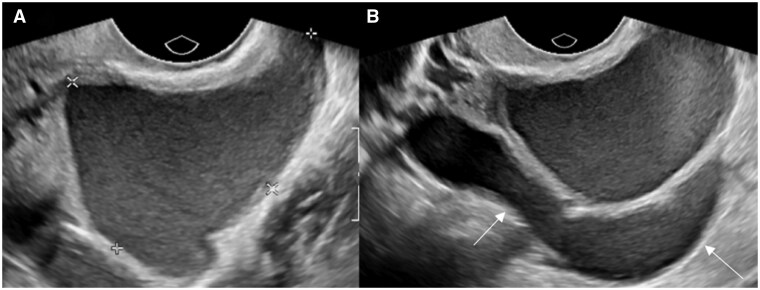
Transvaginal ultrasound of a 51-year-old female showing (A) a left ovarian unilocular cyst with ground glass internal echoes consistent with an endometrioma (with contour deformity related to probe pressure) (B) Haematosalpinx was also noted.
